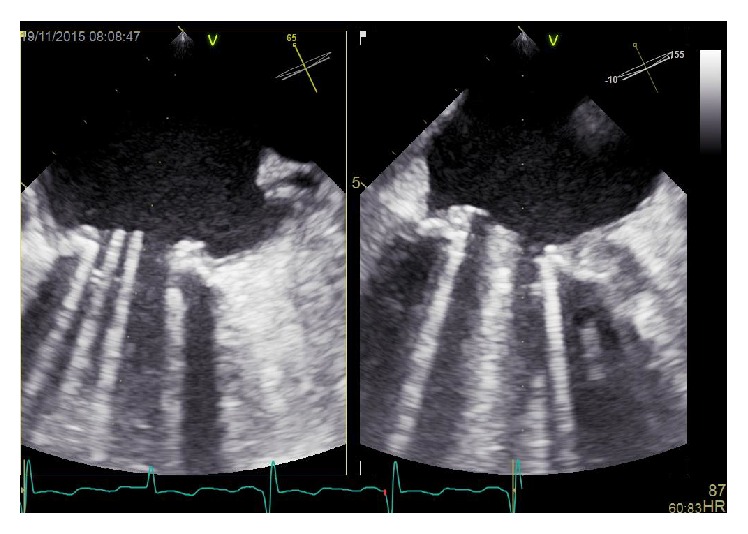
Transesophageal echocardiography of the mitral valve prosthesis after thrombolysis. The thrombus is no longer visible. Normal movement of the disks of the bileaflet valve is visible.
